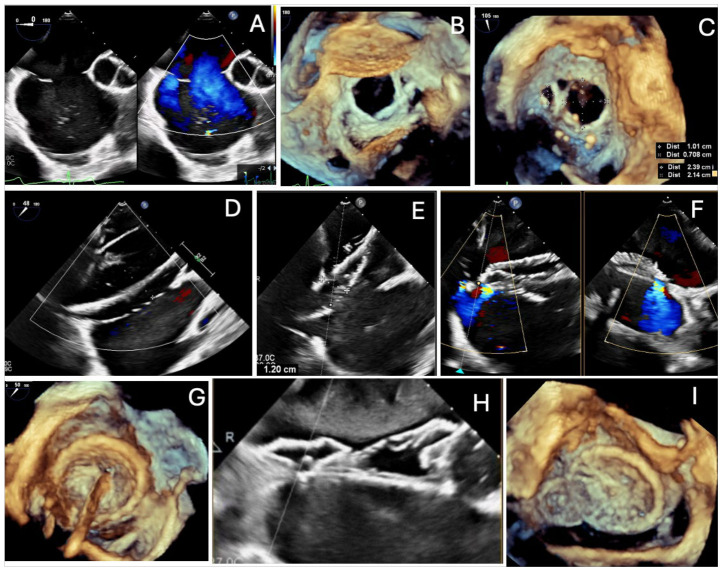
ASD with multiple defects. Image (A), 2D TOE with colour Doppler showing two defects. Images (B,C) are en face LA and RA views respectively, with direct 3D measurements of the two largest defects. Images ((D,E) show two wires placed through each larger defect and balloon sized using stop flow technique (larger defect 30 mm, smaller defect 12 mm). A 33 mm Figula Flex II Occlutech (Occlutech ASD Occluder; Occlutech, Helsingborg, Sweden) device deployed first and not released, images (F,G). Then 13.5 mm device was deployed with careful adjustments to attempt to interlock the devices. Images (H,I) show deployed devices after release.
